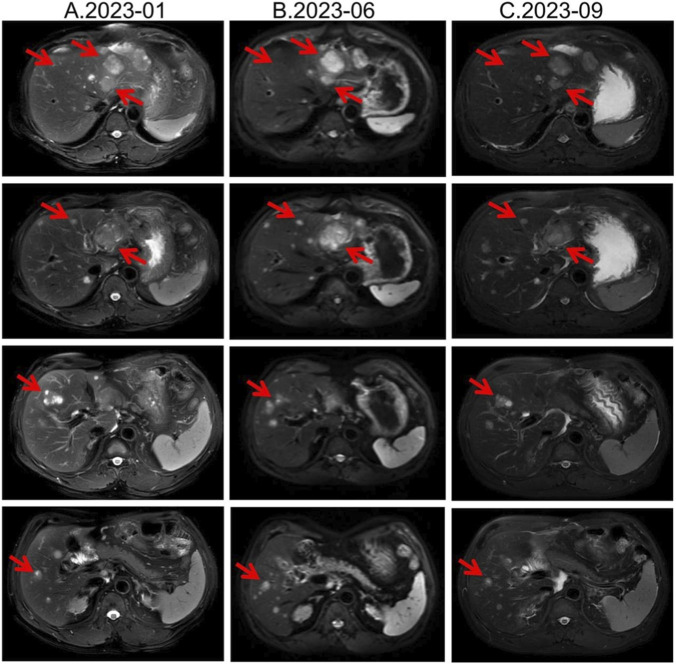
Lesion changes in patients treated with second-line surufatinib. (A) 2023-01 MRI: Multiple enhanced nodular shadows in the liver, with the larger ones located in the left lateral lobe of the liver, approximately 4.4 cm × 3.5 cm in size. Treatment response evaluation: SD. (B) 2023-06 MRI: Multiple enhanced nodules in the liver, with the larger ones located in the left lateral lobe of the liver. Treatment response evaluation: SD. (C) 2023-09 MRI: Multiple enhanced nodules in the liver, with the larger ones located in the left lateral lobe of the liver. Treatment response evaluation: SD.
